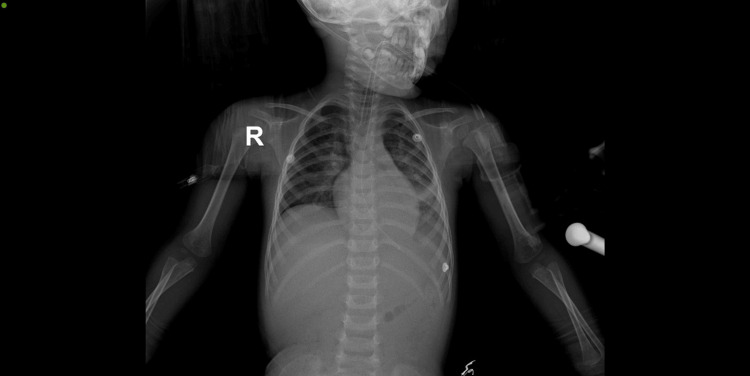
Chest X-ray Bilateral para-hilar opacities more pronounced in the left lung
